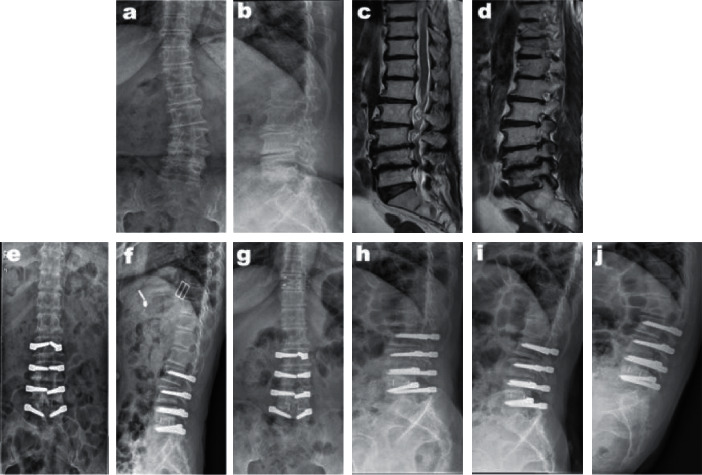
A 49-year-old woman had vertebral canal stenosis at L2–5 and left lateral recess stenosis at L4-5 with DLS (a–d). She underwent fenestration decompression at L3-4, TLIF at L4-5, and instrumentation at L2–5 using the Dynesys system. Postoperative radiographs showed scoliosis correction (e, f), the radiographs obtained 42 months after the operation showed motion preservation and no progression of scoliosis (g–j).
